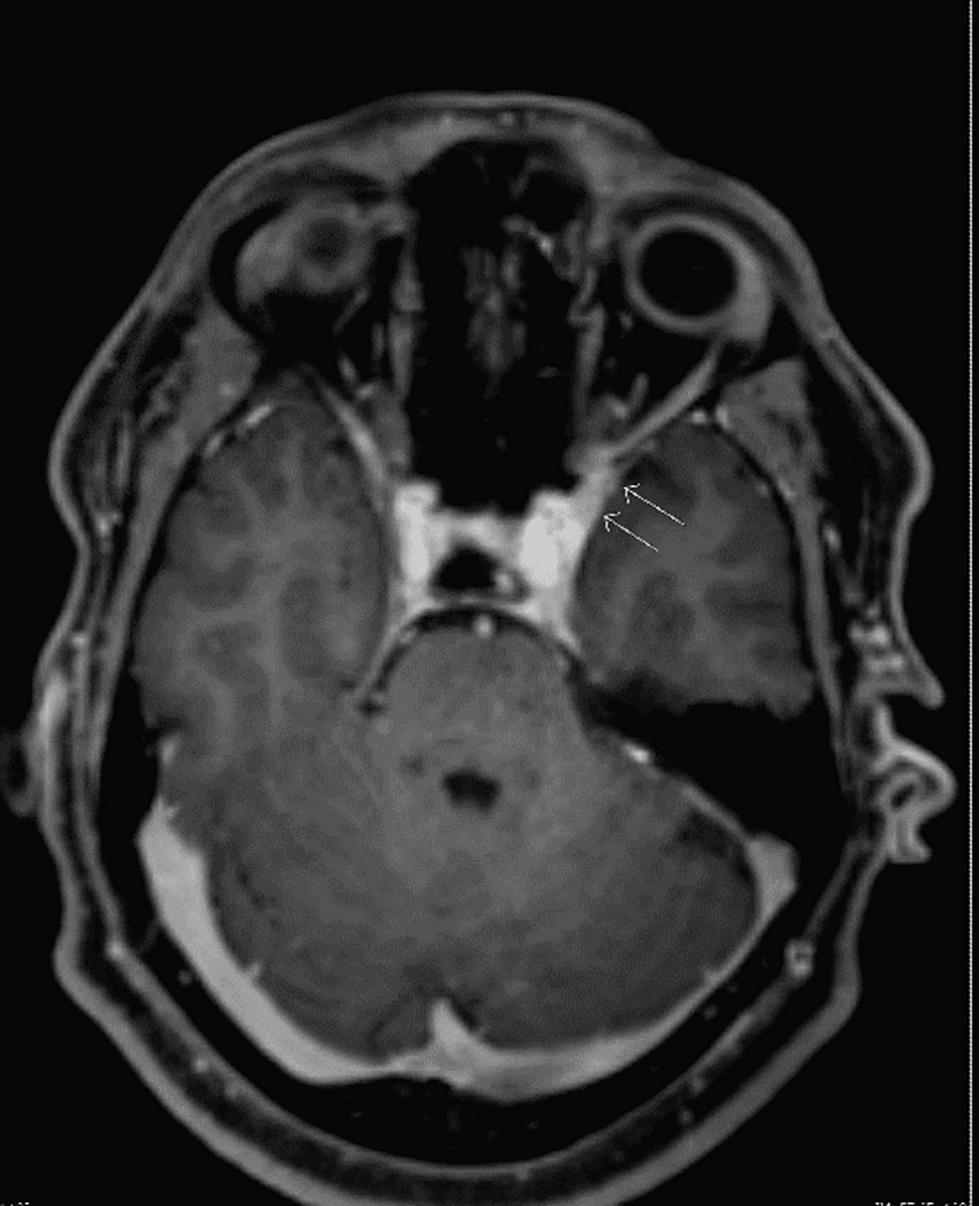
MRI of the brain in T1 view with contrast enhancement showing mild asymmetry of the cavernous sinus regions with relative fullness on the left side and focal lateral thickening. MRI = magnetic resonance imaging
